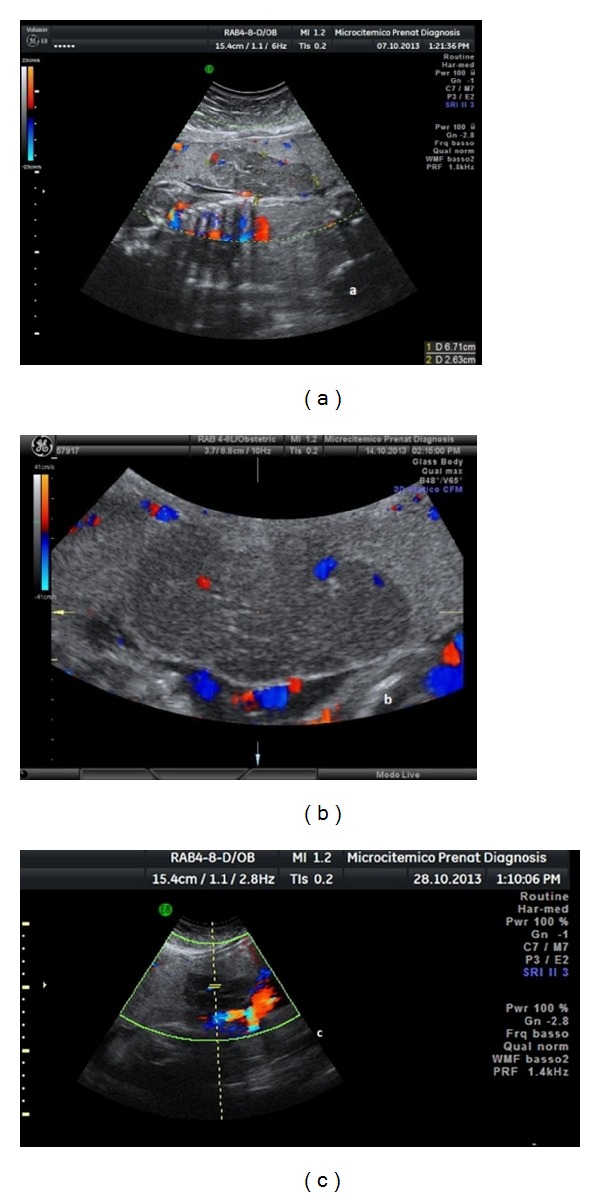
(a) At ultrasound the mass was hypoechogenic, sharply marginated, and circumscribed by a hyperechoic capsule, from which numerous intralesional septa appeared to originate, with some anechoic central areas of vasculature and peripheral vessels (the feeding vessel). Score assigned is 3. (b) Successive examination score assigned is 3. (c) Score assigned is 2.
